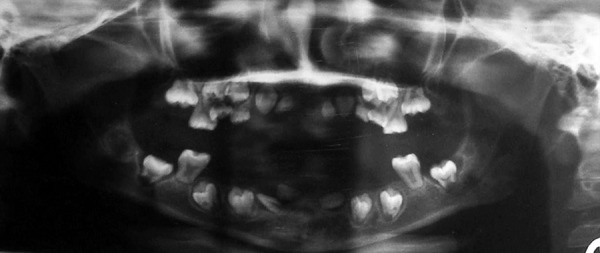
Orthopantomogram shows typical appearance of teeth affected with DD-I, with underdeveloped roots, narrow pulp chambers and periapical radiolucencies
